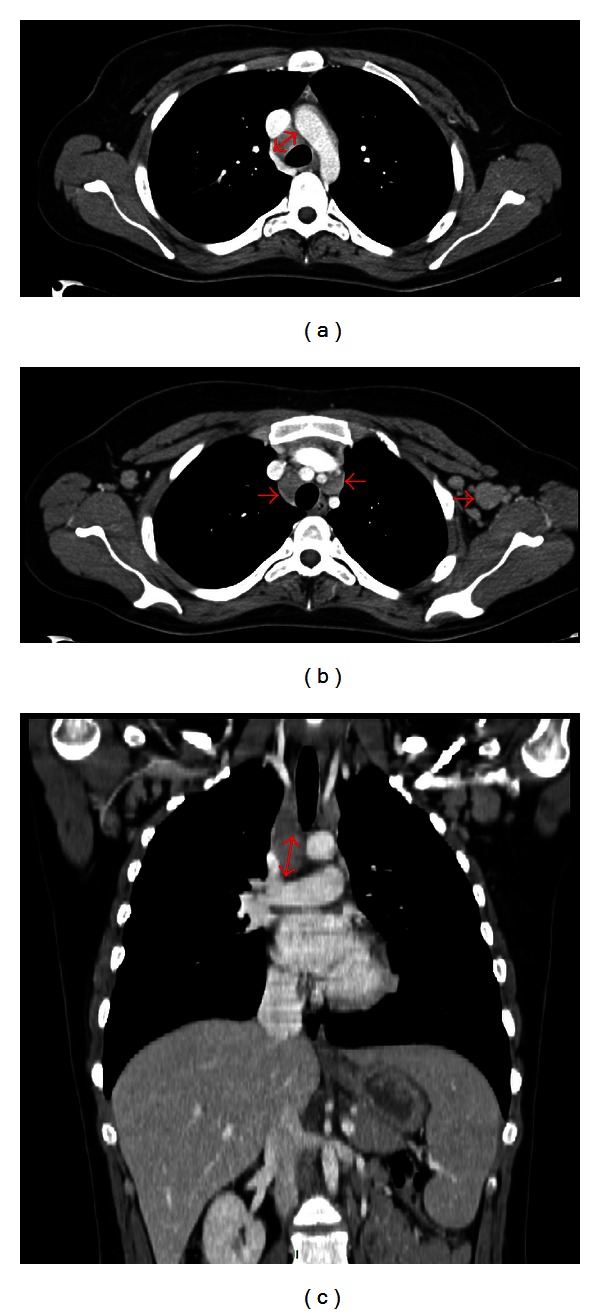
Computed tomography scan showed mediastinal and axillary lymph nodes up to 20 mm in diameter on axial ((a) and (b); red arrows) and coronal ((c); red arrow) slices.
